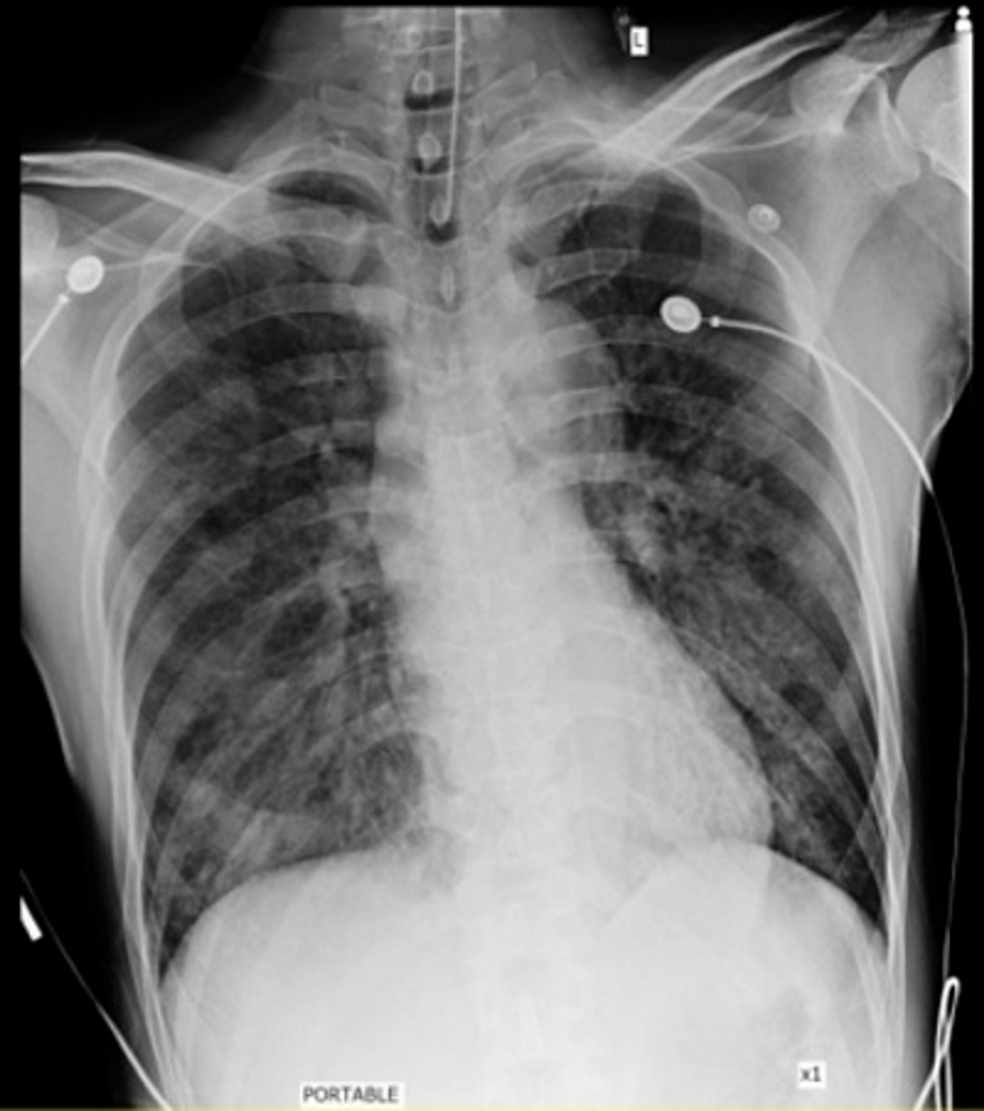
The patient’s chest x-ray shows moderate pulmonary edema
